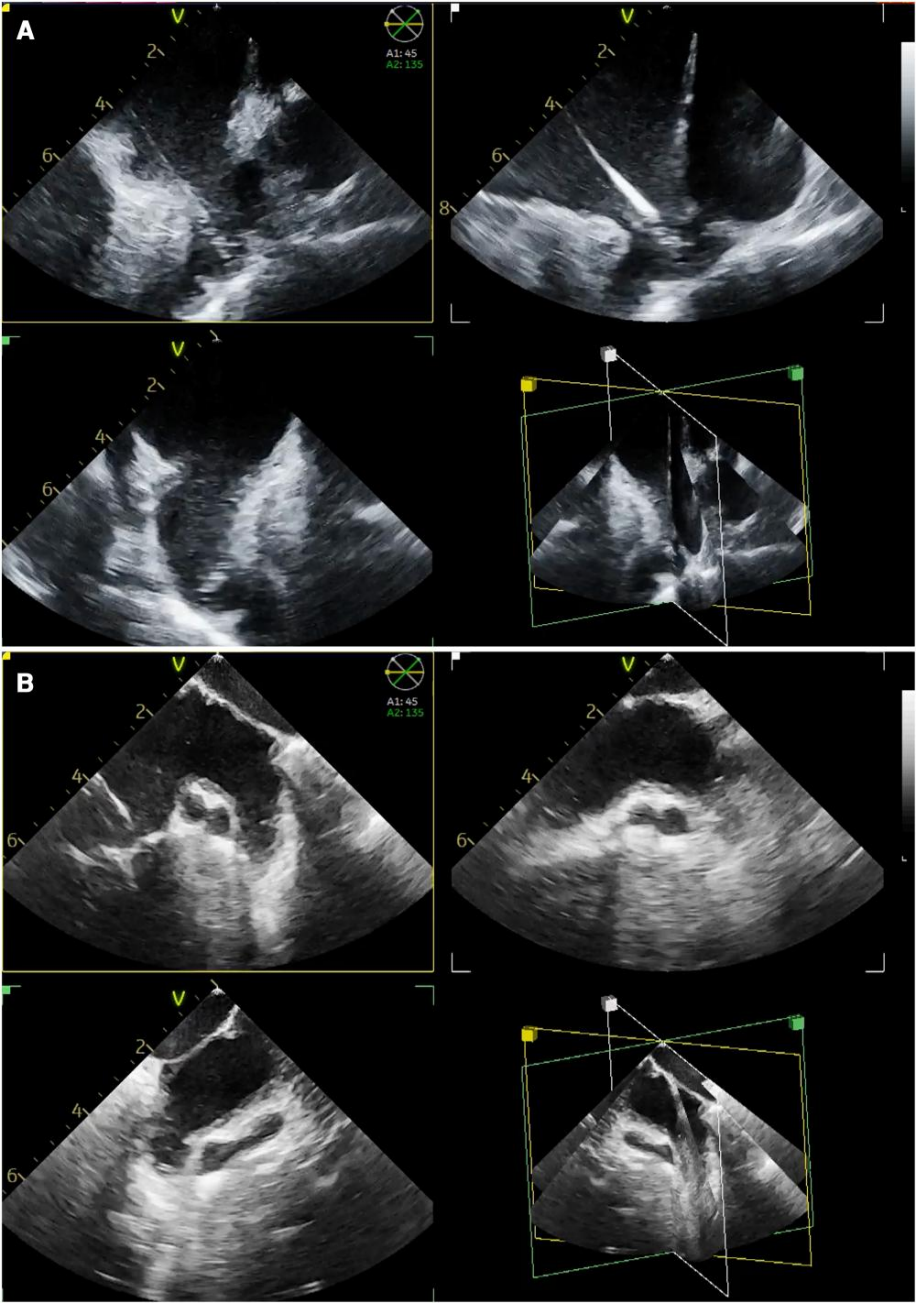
Periprocedural MPRs to assess LAA morphology and size in two patients (panels *A* and *B*). 3D-ICE MPRs (45°–90°–135°) for LAA sizing before occlusion. The Watchman sheath can be seen in the LA over pigtail wire in panel *A*. Multiplanar reconstruction allows for scanning and sizing the LAA from different angles without the need to reposition the ICE probe. ICE, intracardiac echocardiography; LAA, left atrial appendage; MPR, multiplanar reconstruction.
